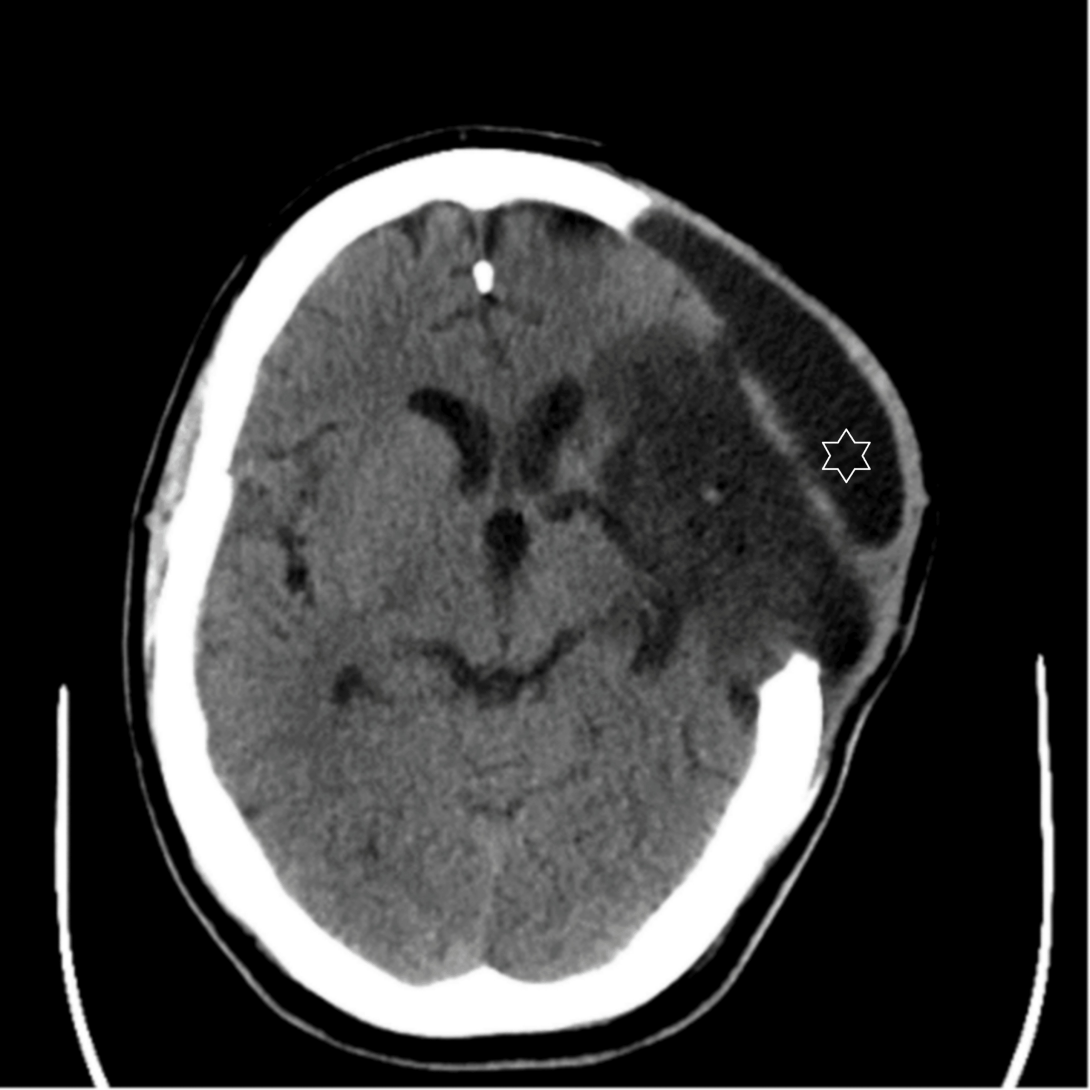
CT brain plain-axial images. Post craniectomy changes and established left MCA territory infarct. Overlying fluid density collection with a maximum thickness of 20 mm suggestive of Pseudomeningocele
